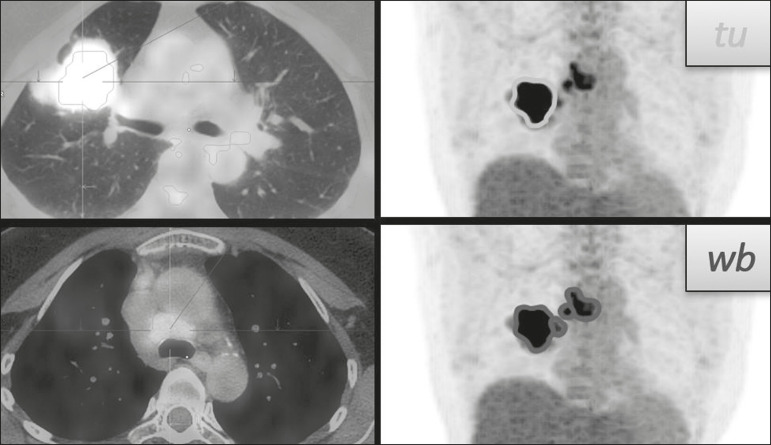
^18^F-FDG PET/CT in a patient with advanced NSCLC, showing the VOIs for the tuTLG (upper) and wbTLG (lower).
